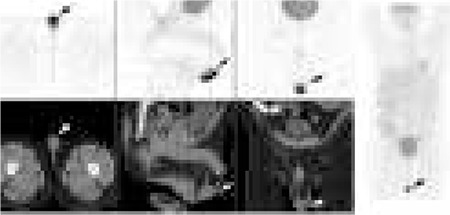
Intense FDG uptake in the left testis and the inferior left scrotal sac (arrow)
